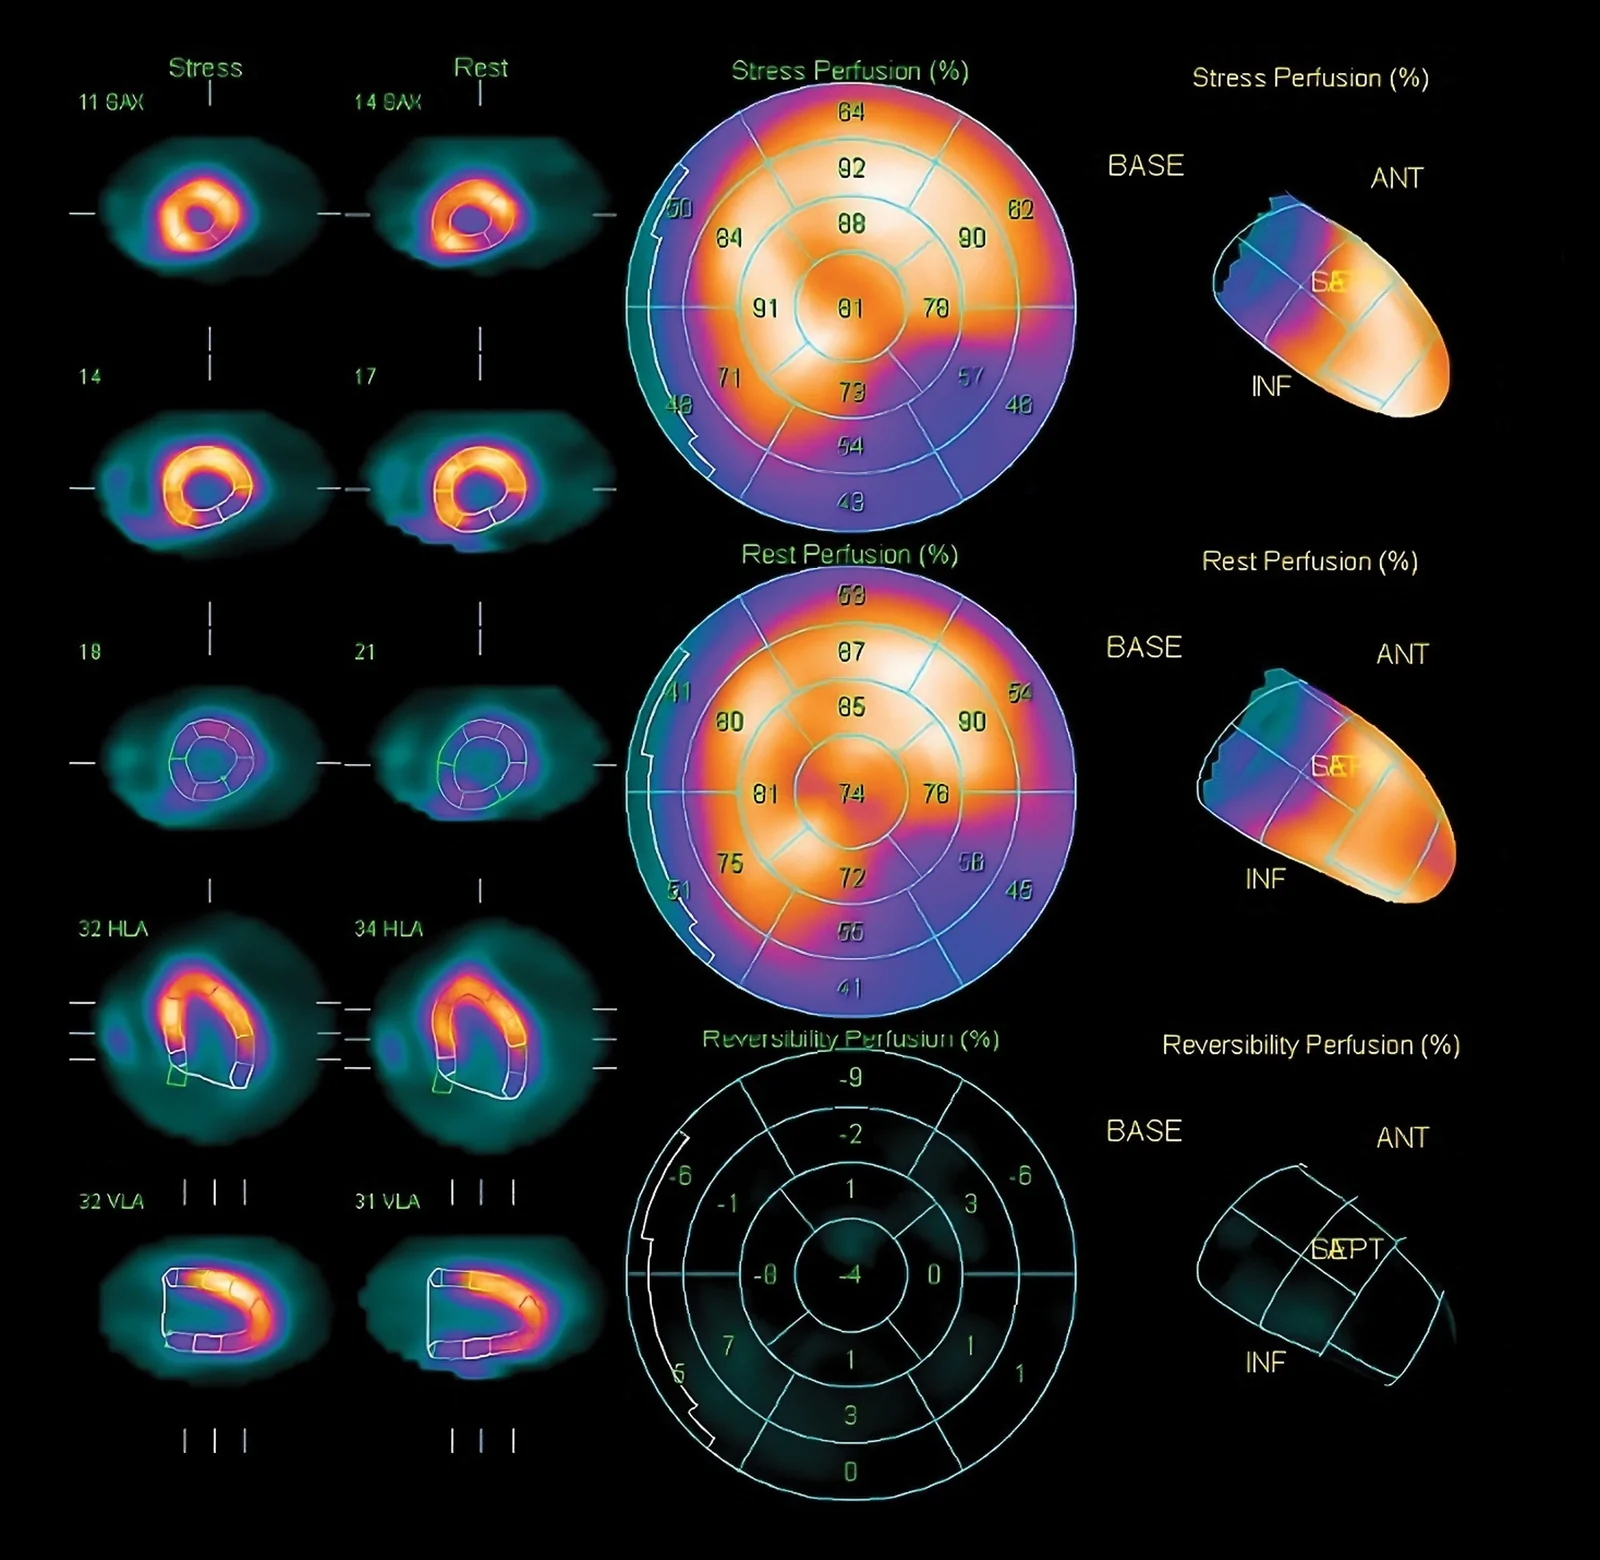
Six-month follow-up myocardial perfusion scintigraphy after coiling, showing resolution of inferolateral hypoperfusion with no evidence of stress-induced ischaemia.
